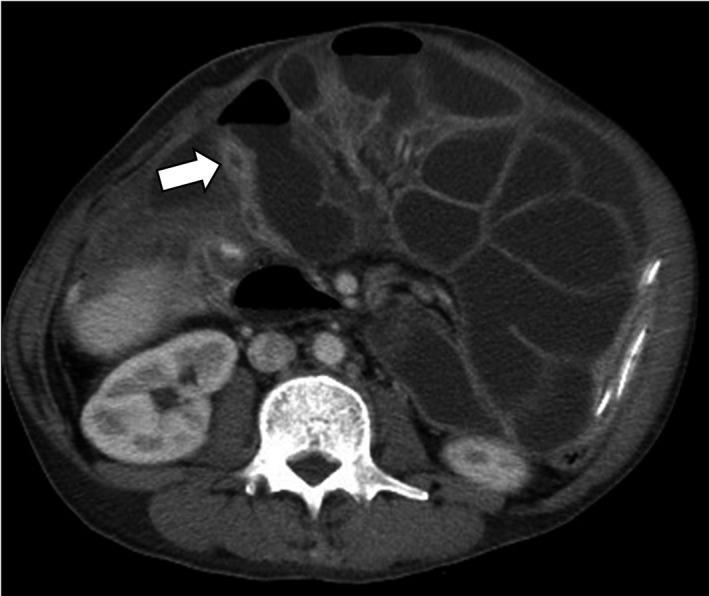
Abdominal computed tomography showing the dilatation and collapse (arrow) of the small intestine and partial thickening of the bowel wall (arrow)
